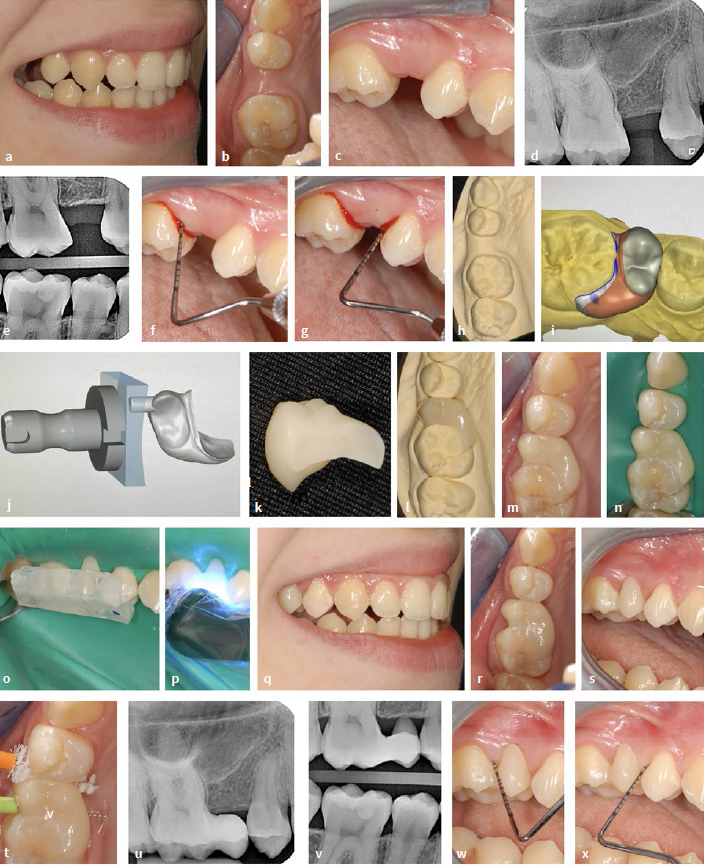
Indirectly (extraorally) fabricated workpiece (digitally fabricated) using the example of a category II NPB in a 21-year-old female patient. (a) to (c) Initial clinical situation with gap (upper right second premolar) of premolar width. (d) and (e) Initial radiographic situation. (f) and (g) bleeding on probing on the tooth surfaces facing the gap. (h) Plaster model. (i) to (k) Digital design of the pontic. (l) Try-in on the model. (m) Try-in in the mouth. (n) Try-in under rubber-dam. (o) Adhesive insertion with individual fixation key (placing guide). (p) Light curing. (q) to (s) Clinical situation after gap closure. (t) Try-in of matching interdental brushes. (u) and (v) Radiographic situation after gap closure. (w) and (x) Check after 2 months, no pathological findings, no bleeding on probing.
